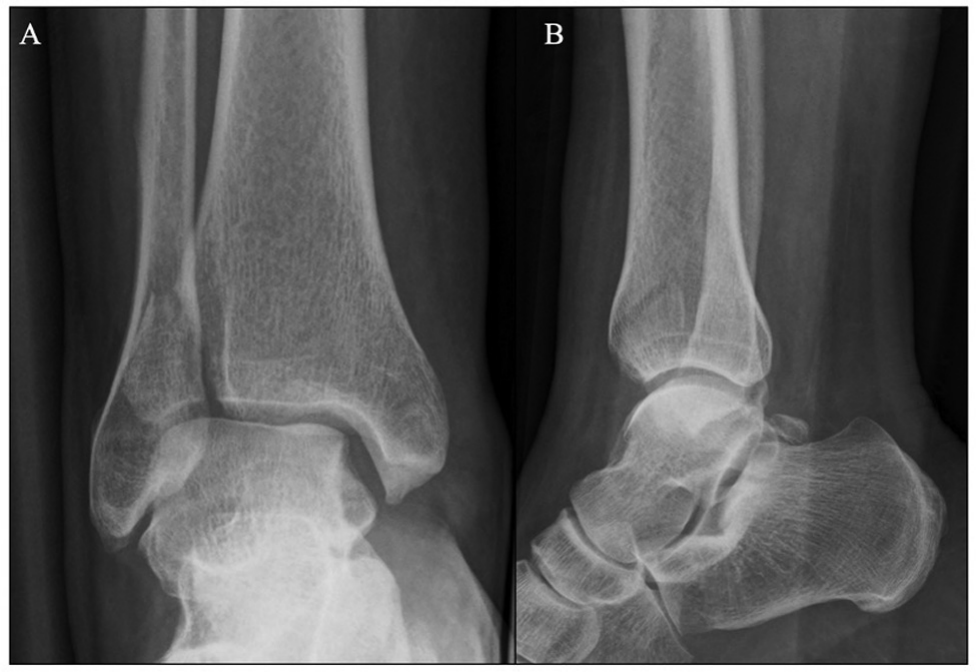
Preoperative X-ray view: (
